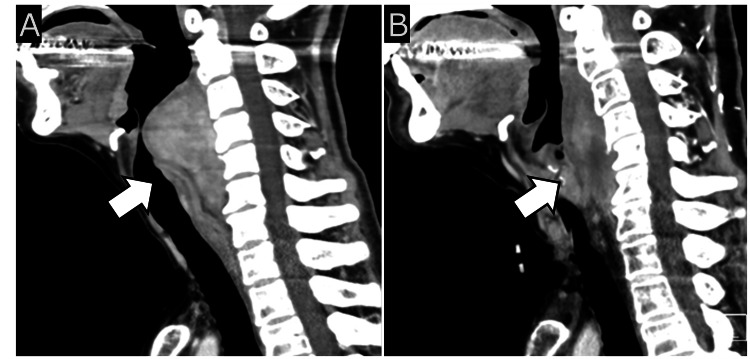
Pre- and post-treatment CT findings. (A) Pre-treatment CT: Sagittal contrast-enhanced CT image showing a large, irregularly enhanced mass (white arrow) occupying the retropharyngeal and danger spaces, extending to the thyroid level.
